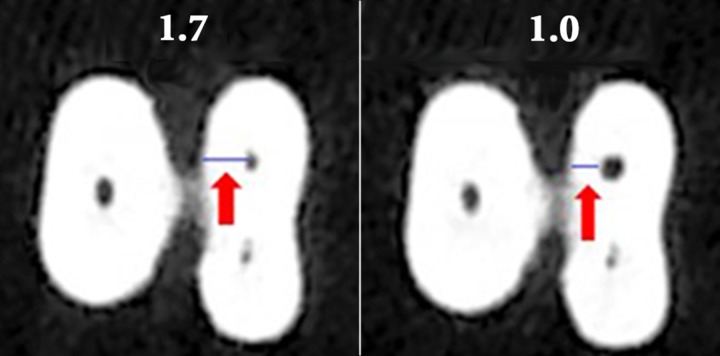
Dentin thickness measured in the danger zone area in CBCT images obtained before (left) and after (right) preparation
